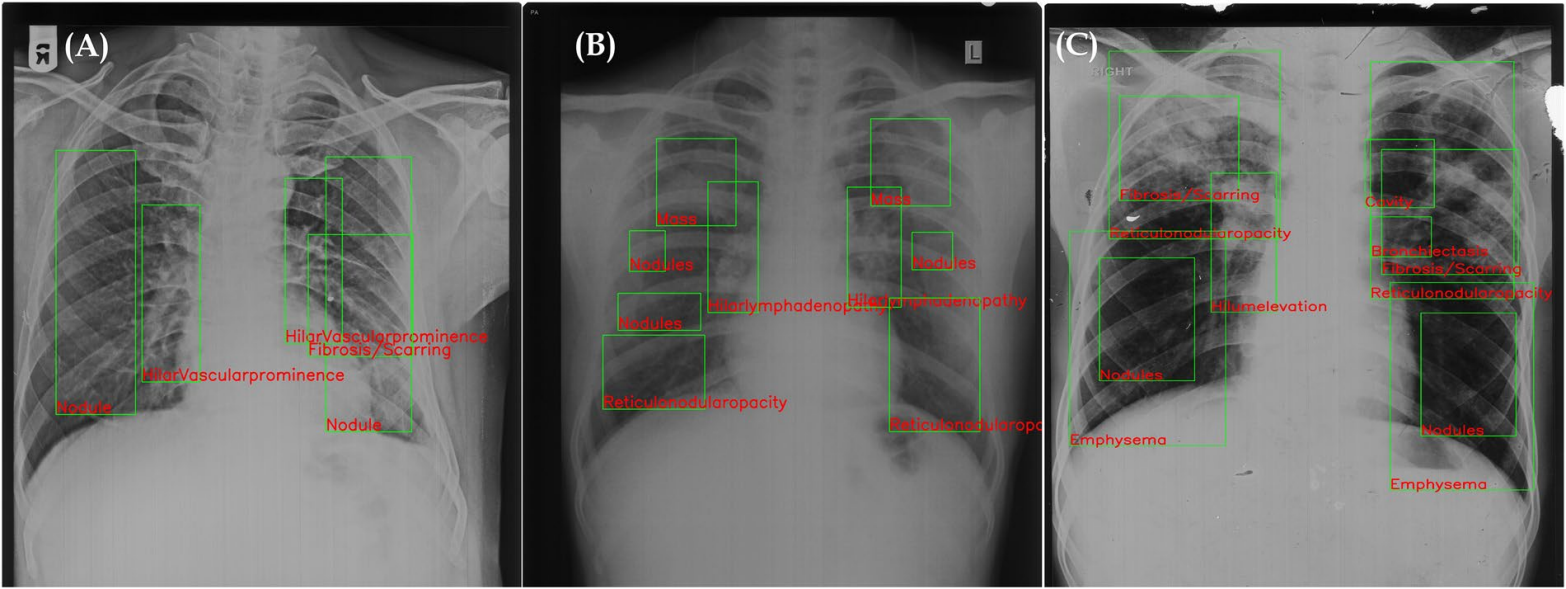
Sample chest X-rays of individuals with (A) Silicosis, (B) Silicotuberculosis, and (C) Tuberculosis, highlighting different manifestations present for three disorders.
